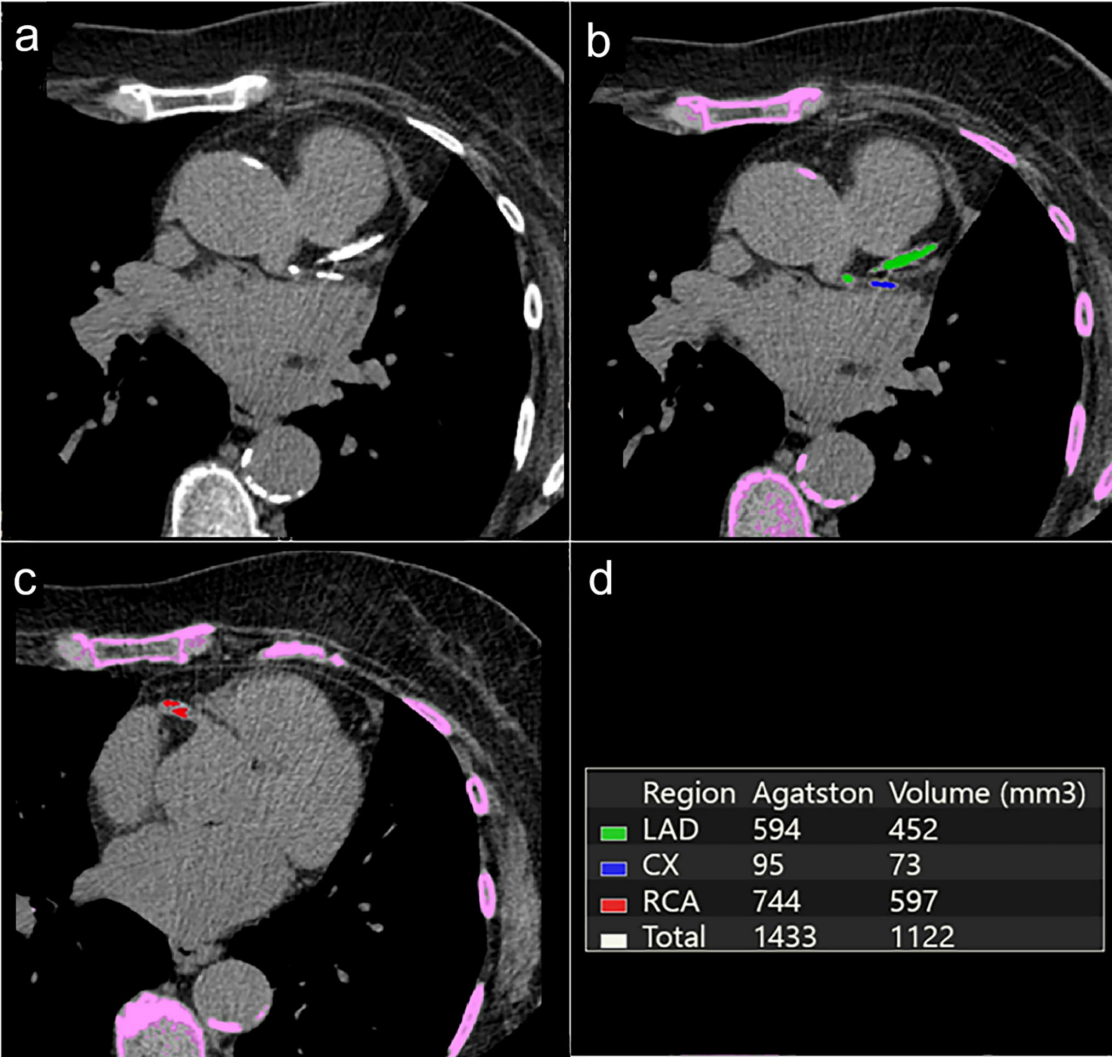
Coronary Calcium Scoring. Non-enhanced CT image showing coronary calcifications on proximal LAD and CX (a) followed by color-coded trans-axial images that highlights moderate calcifications on proximal LAD, CX (b) and RCA (c). Agatston Score is calculated to quantify the extent of coronary calcium (d). CT, computed tomography; LAD, Left Anterior Descending artery, CX, Circumflex Artery; RCA, Right Coronary Artery
